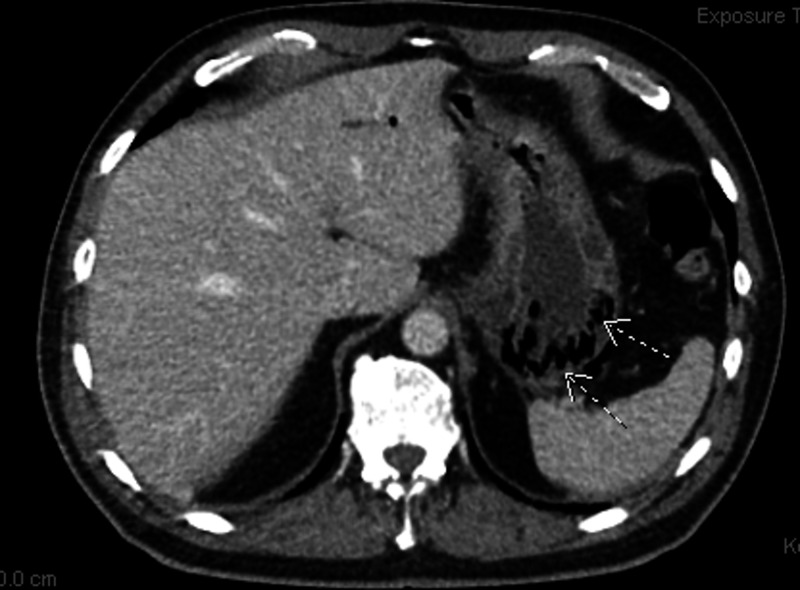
CT scan axial view showing gas in the stomach walls CT: computed tomography
